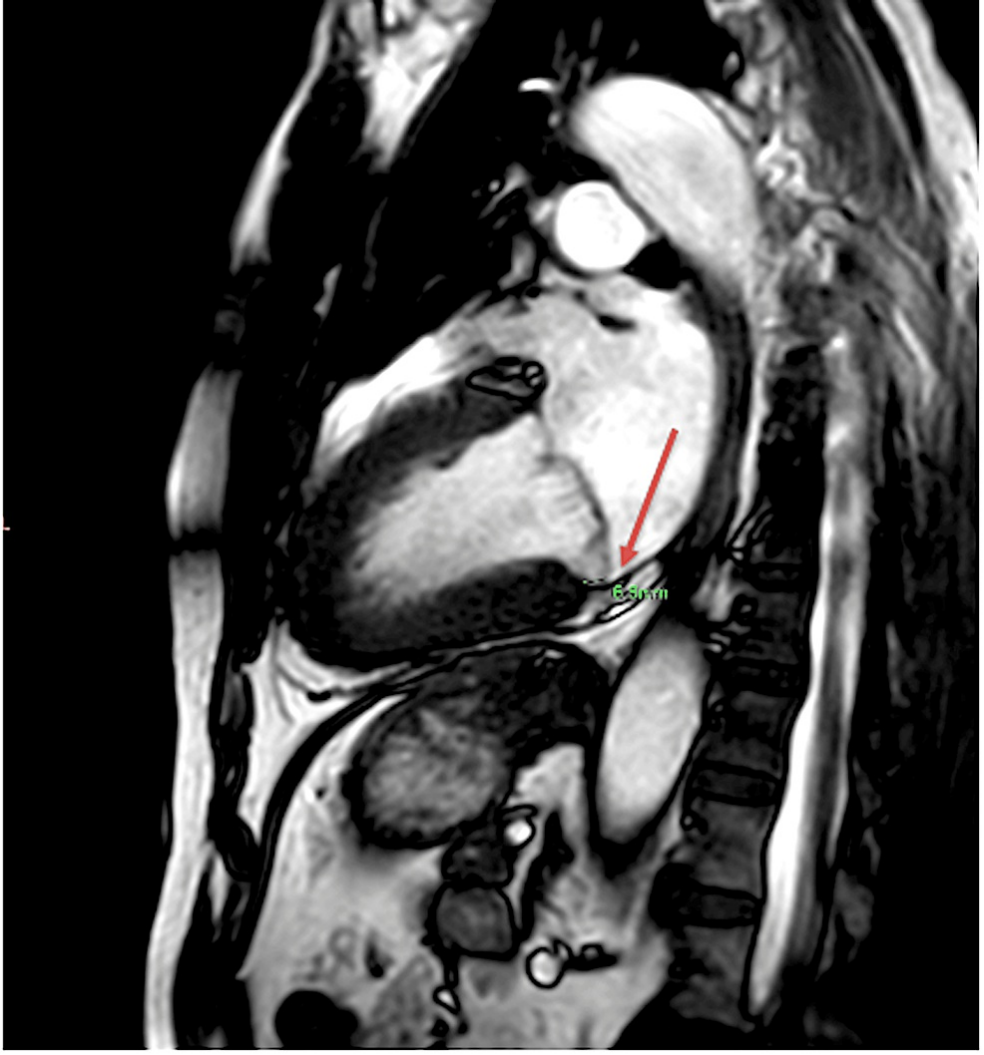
Cardiac MRI with an arrow indicating mild mitral annular disjunction (6.6 mm) MRI: magnetic resonance imaging
